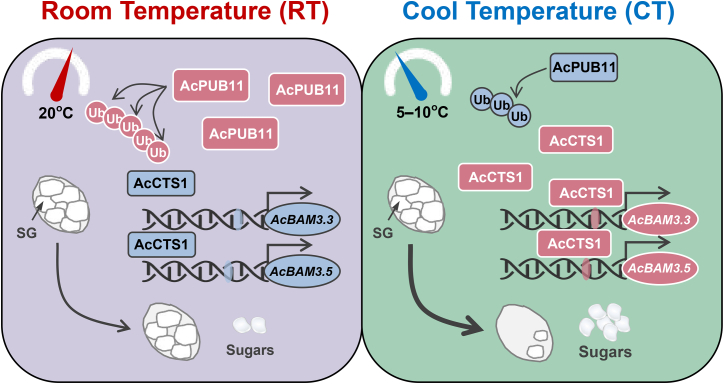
Proposed regulatory network of cool temperature-induced starch degradation in kiwifruit. At RT, AcPUB11 interacts with and ubiquitinates AcCTS1, leading to the proteasomal degradation of AcCTS1; this represses AcCTS1-mediated induction of *AcBAM3.3* and *AcBAM3.5*, resulting in stable starch content in the fruit. When kiwifruit are ripened at CT, reduced temperature decreases AcPUB11 abundance, thereby increasing AcCTS1 abundance. Consequently, AcCTS1 directly targets and activates the expression of *AcBAM3.3* and *AcBAM3.5*, leading to starch degradation and a subsequent increase in fruit sugar content. SG, starch granule.
